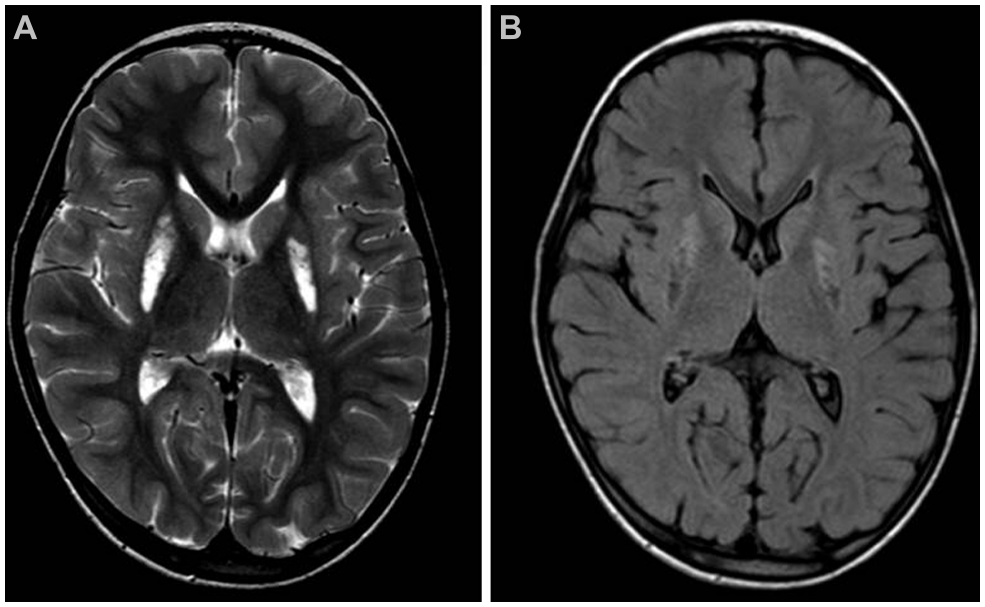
Brain MRI. Axial T2-weighted (A) and FLAIR (B) images of the patient, taken at 7 years of age. Note the hyperintense signals due to gliosis (A) and the cavitations (B) in the lentiform nuclei.
